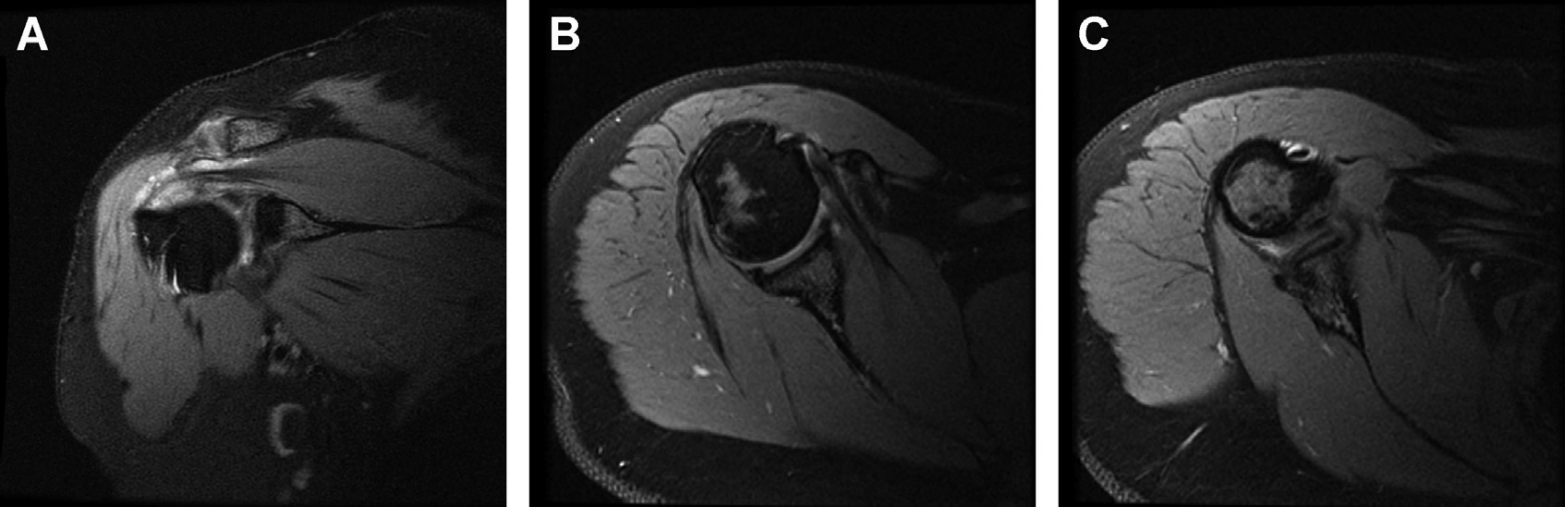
Proton dense fat saturated MRI images from case two showed partial-thickness tears of the supraspinatus, infraspinatus (A. coronal oblique section), and subscapularis (B. axial section) on a background of underlying tendinosis. (A) There was evidence of subacromial bursitis as well. (C) On the axial cut, biceps tendonitis is apparent.
